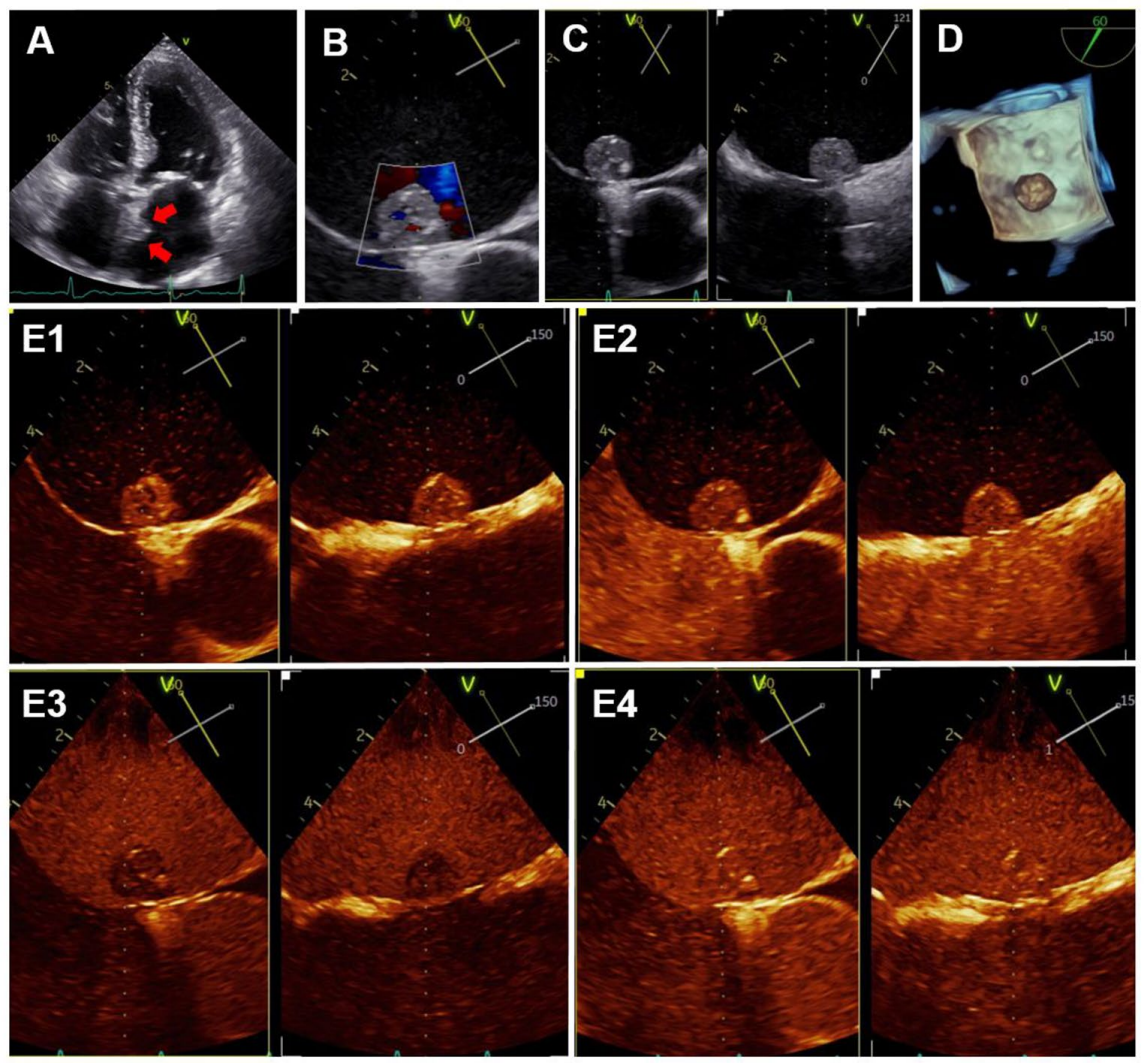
A transthoracic echocardiography in an 83-year-old woman revealed a thickening of the intra-atrial septum/mass in the LA (A). Further diagnostic via transesophageal echocardiography confirmed a 15 × 14 × 14 mm ball-shaped echo-inhomogeneous left-atrial mass originating from the intra-atrial septum without infiltration of adjacent structures (Panels C and D). *Worm-like* anechoic structures beside echo-dense areas and color-Doppler evaluation suggested blood vessels (Panel B). Intravenous SonoVue (Bracco Imaging) echo contrast agent was applied. It showed distribution from the right heart (E2) to the left atrium, initially sparing the intra-atrial septum mass (E3), before it adapted its echogenicity to the left atrial blood pool (E4)(Movie in data supplement).
